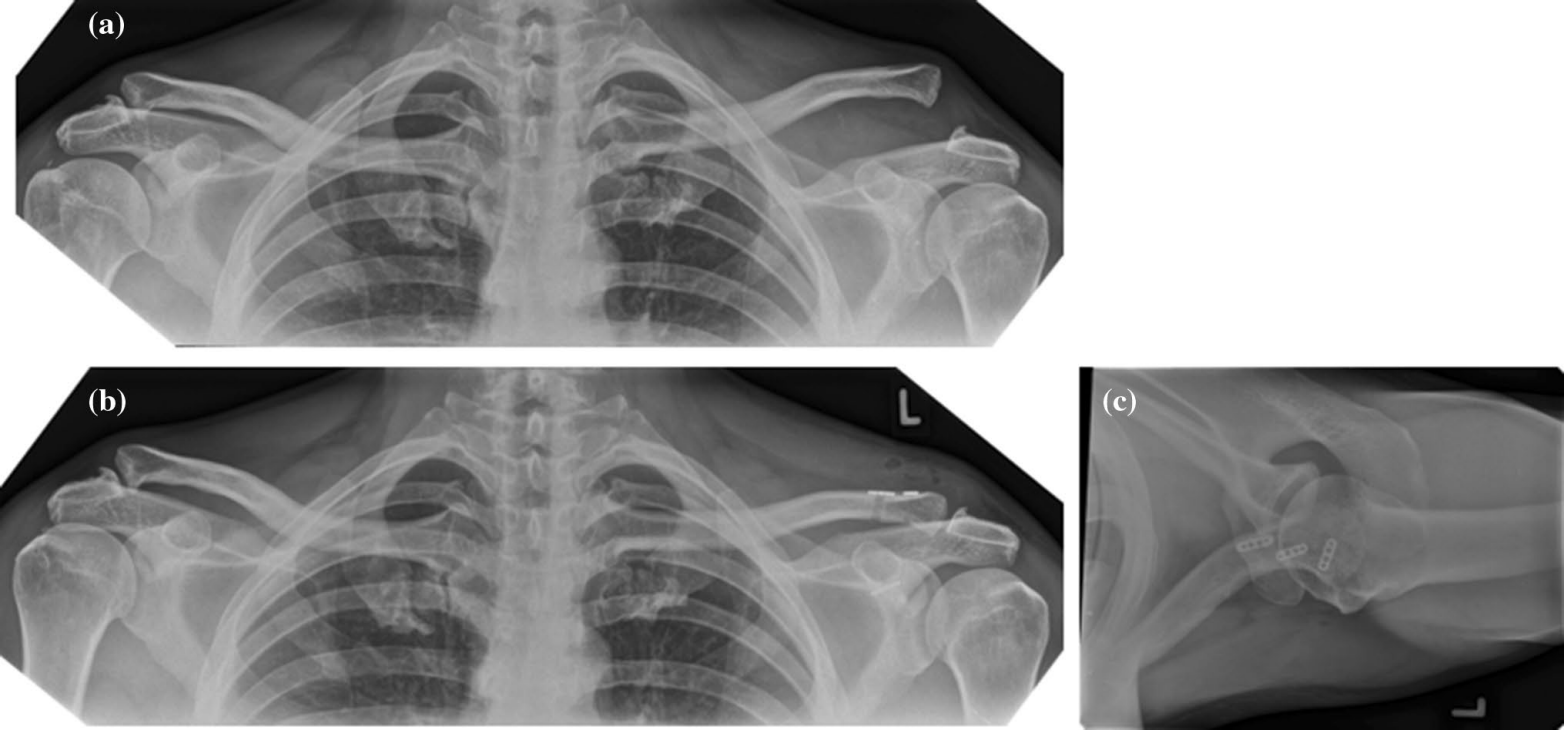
A, B, C Stress radiographs before and after reconstruction and axial view confirming horizontal alignment
